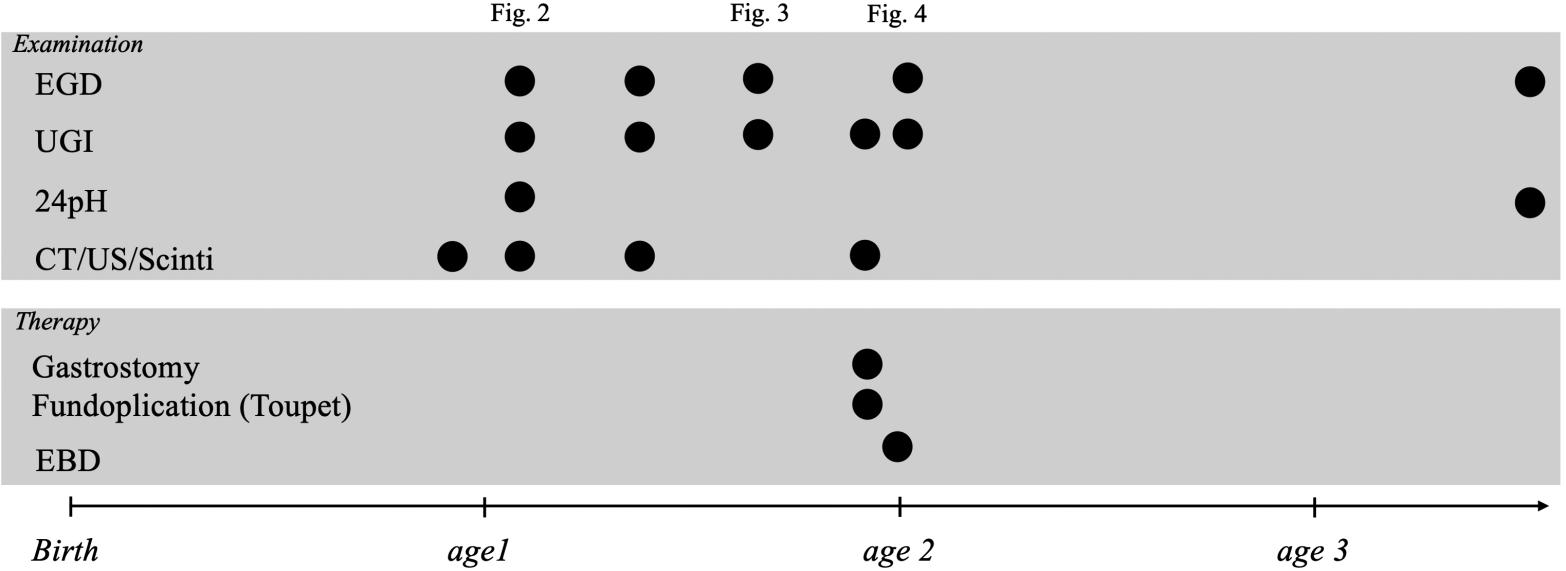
Patient's clinical course, including examinations and invasive treatments. EGD, esophagogastroduodenoscopy; UGI, upper gastrointestinal series; CT, computed tomography; US, ultrasonography, EBD, endoscopic balloon dilation.
